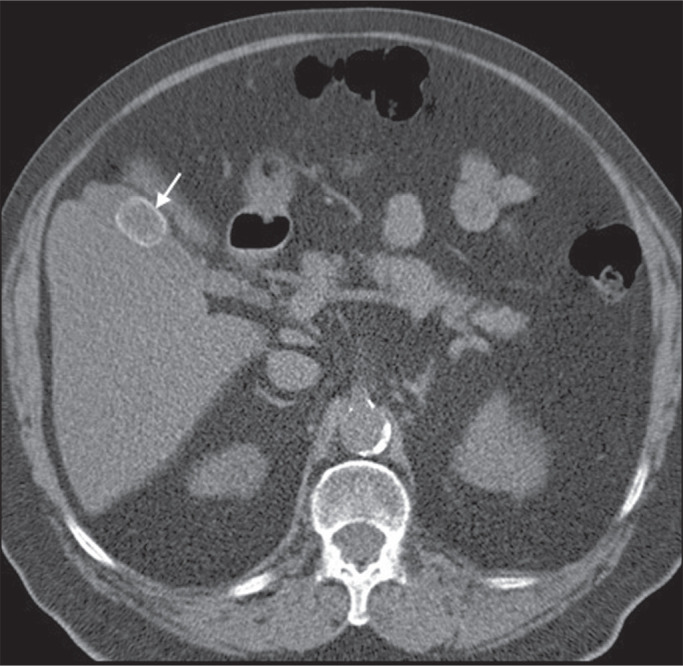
Unenhanced axial CT image showing a gallstone (arrow) in a patient
